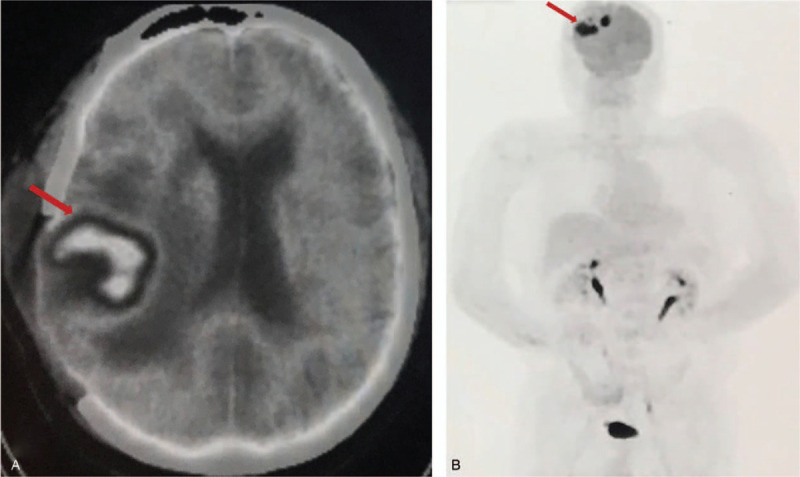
The ^18^FDG PET-CT scan showed significant FDG uptake in the right frontal and parietal lobes (arrow) combined brain edema (A) and no significantly increased metabolic activity in other regions (B). PET-CT =positron emission tomography-computed tomography.
